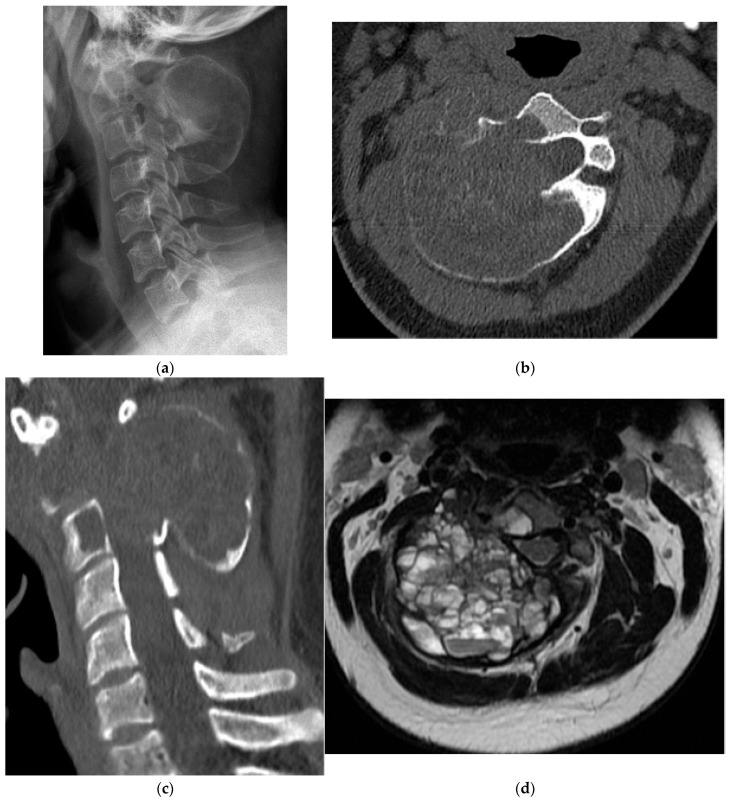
Aneurysmal bone cyst. (a) Large lucent expansile mass centered in the posterior elements of C2 and C3. (b) Large expansile mass in the posterior element of the C3 vertebral body. There is thinning of the cortex. (c) Sagittal CT bone window: the mass involves the posterior elements and the vertebral bodies of C2 and C3. (d) Axial T2 MR, multiple fluid–fluid levels representing serum hematocrit levels of intralesional blood.
